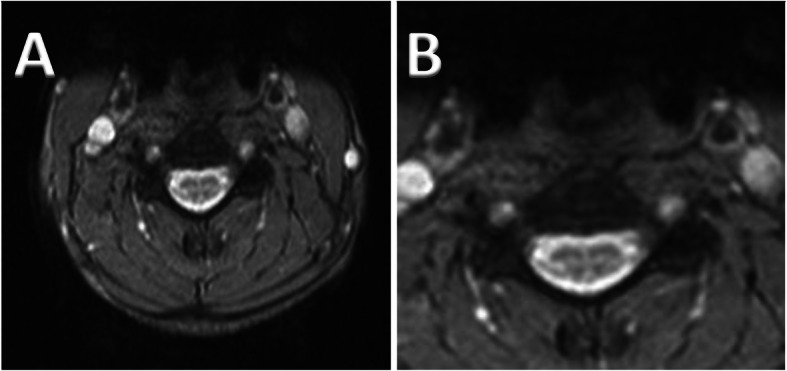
Axial T2 MRI spinal images (Patient 4) showing signal changes affecting mainly lateral Corticospinal tracts (magnified in image b)
